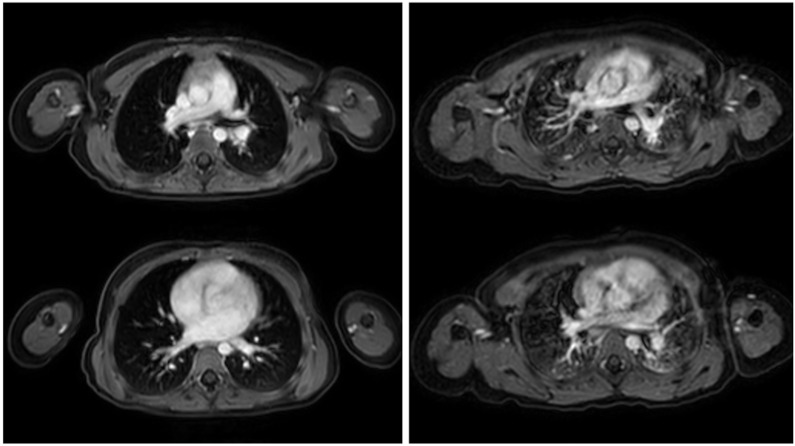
Side-by-side comparison of a post-contrast T1-weighted sequence acquired with a navigator-based technique (left) and a post-contrast T1-weighted sequence obtained during breath-hold (right), demonstrating respiratory motion artifacts in the latter.
